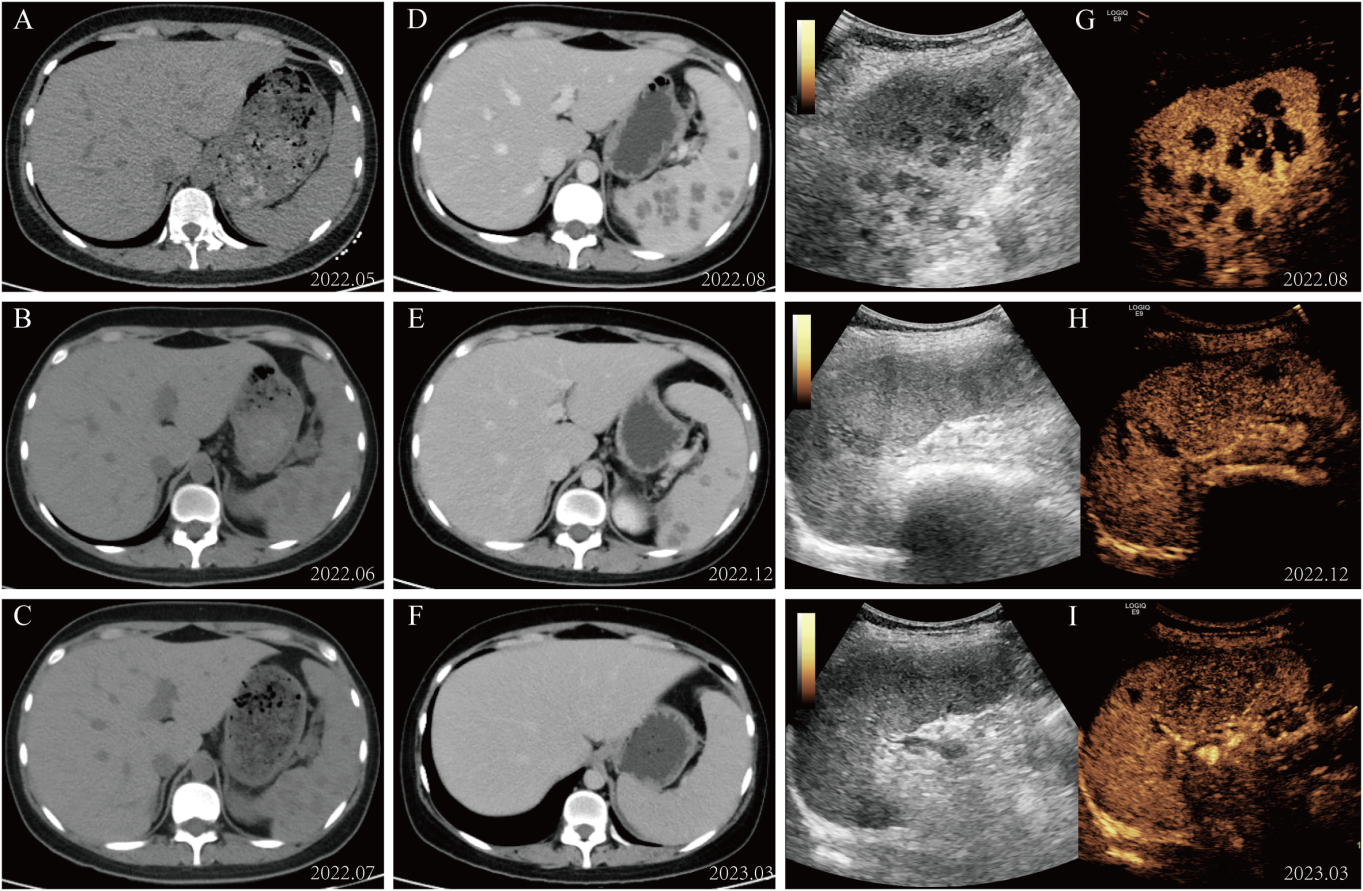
Imaging alterations during patient management. (A–C) The abdominal CT scan revealed diffuse small round low-density lesions in the spleen. (D–F) The splenic lesions exhibited significant resolution following treatment with amphotericin B. (G–I) Changes in contrast-enhanced ultrasound of the spleen during administration of amphotericin B therapy.
